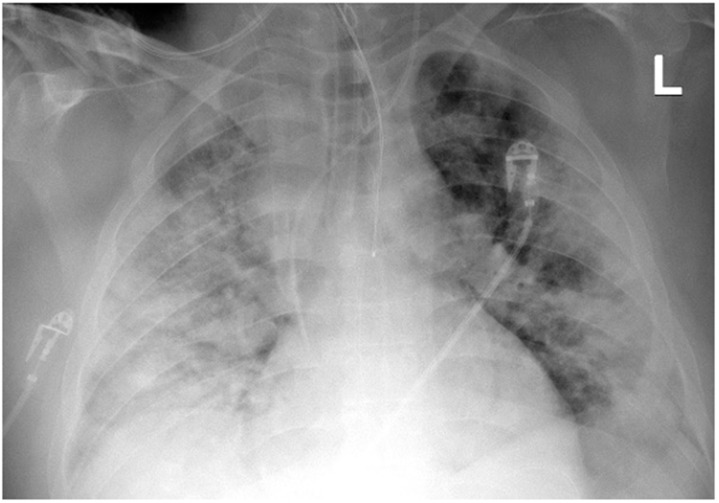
Chest X-ray after insertion of the 21F cannula into the superior vena cava (Case 1).
